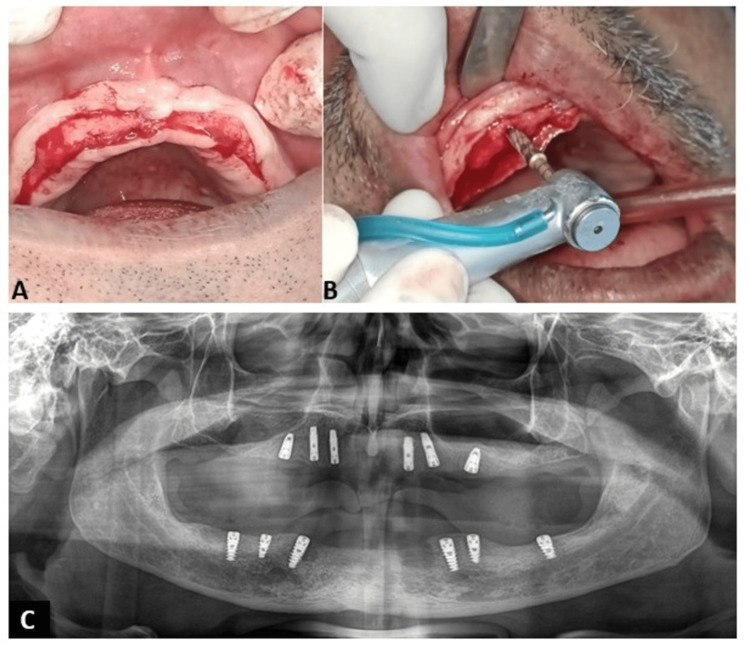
Surgical phase of implant placement and postoperative radiographic evaluation A: Mid-crestal incision in the maxillary arch with reflection of a full-thickness mucoperiosteal flap, exposing the underlying alveolar ridge for implant placement; B: Osteotomy preparation using a Densah bur in densifying mode, demonstrating the osseodensification technique to enhance bone density and primary stability in the maxilla; C: Postoperative orthopantomogram (OPG) showing the position, angulation, and distribution of implants in both arches, confirming adequate anteroposterior spread and avoidance of critical anatomical structures
